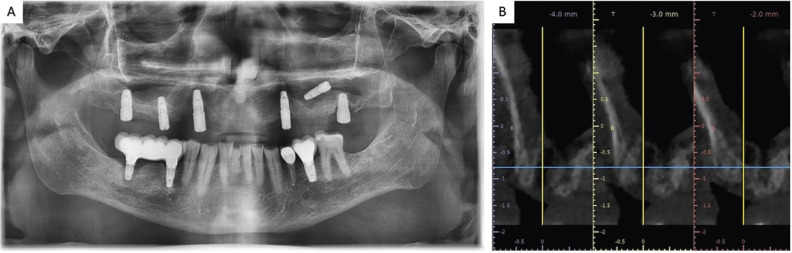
A. Orthopantomography performed intraoperatively, after migration of the implant into the sinus cavity. B. Cone-beam computerized tomography performed before the rescue of the migrated implant.
